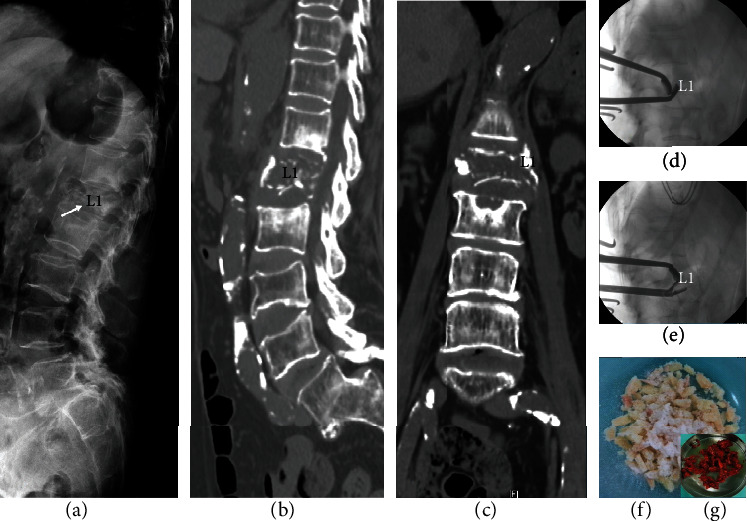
(a) Lateral radiograph and (b) axial and (c) coronal CT reconstructions showed a collapsed nonhealing L1 vertebral body with destruction of both upper and lower endplates. (d, e) T11/T12 and T12/L1 transforaminal debridement using curettes to remove disc material and cartilage. (f) Antibiotic impregnated allograft. (g) Autologous bone graft from posterior iliac crest.
